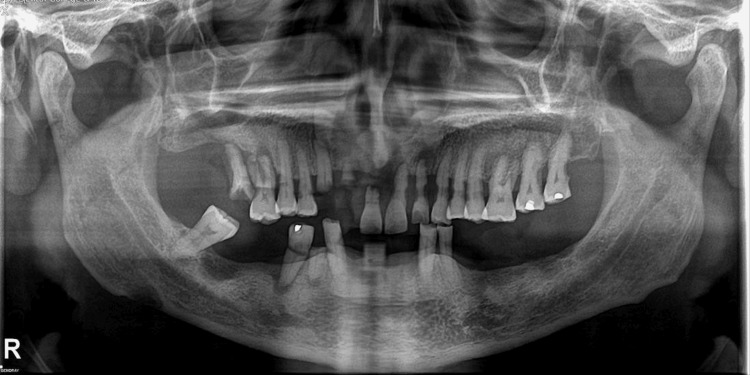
Pre-operative orthopantomograph (Case 2)
